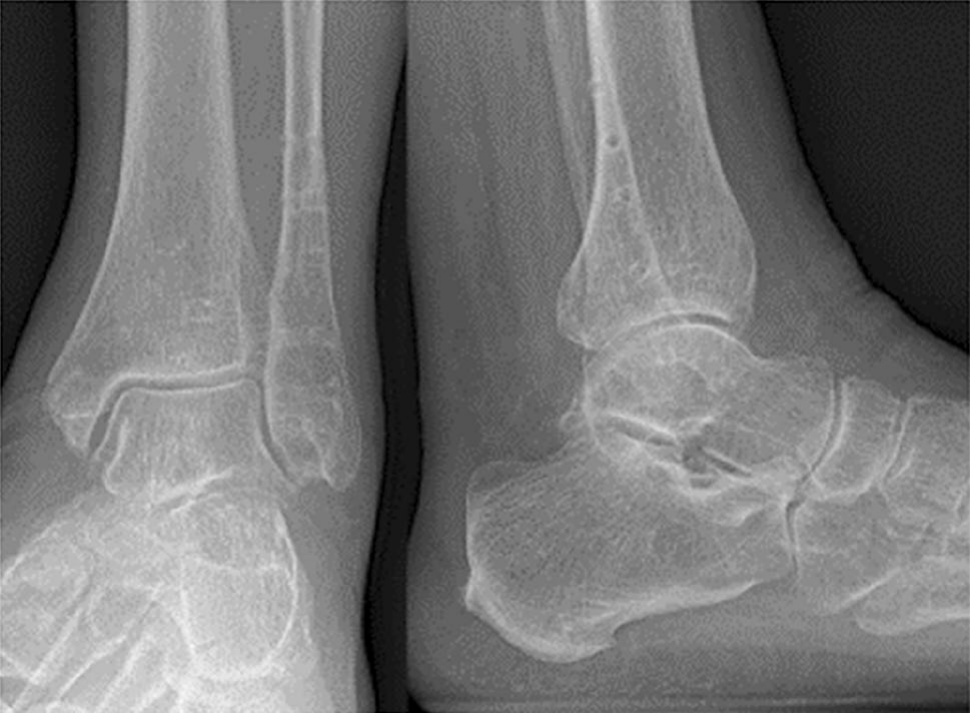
Standing anteroposterior and lateral radiographs at a follow-up of 19 months after the injury and implant removal showing a congruent ankle joint without radiographics signs of posttraumatic arthritis (same patients in Figs. 1,2,3,4)
